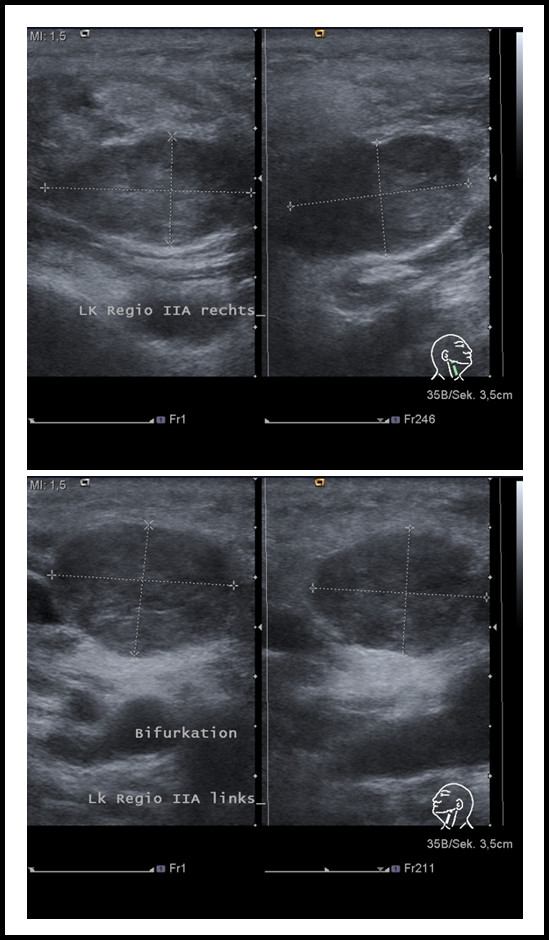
Ultrasound examination of the lymph-nodes in regio IIA on both sides (upper panel: the lymph node on the right side; lower panel: the lymph node on the left side): The masses showed a widely defined border, a hypoechoic internal pattern with anechoic areas and a dorsal enhancement. No vessels within the lesions could be detected by Doppler-ultrasound and the regular lymph node anatomy with hilum and cortex was depleted. Therefore, the lesions were suspicious of containing metastastic tumor masses.
